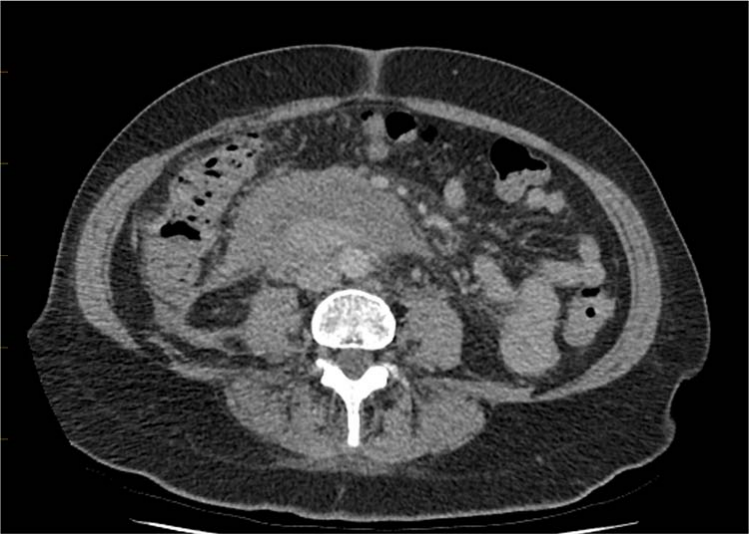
CT abdomen, axial view. Slight interval decrease in size of previously identified periduodenal hematoma.
